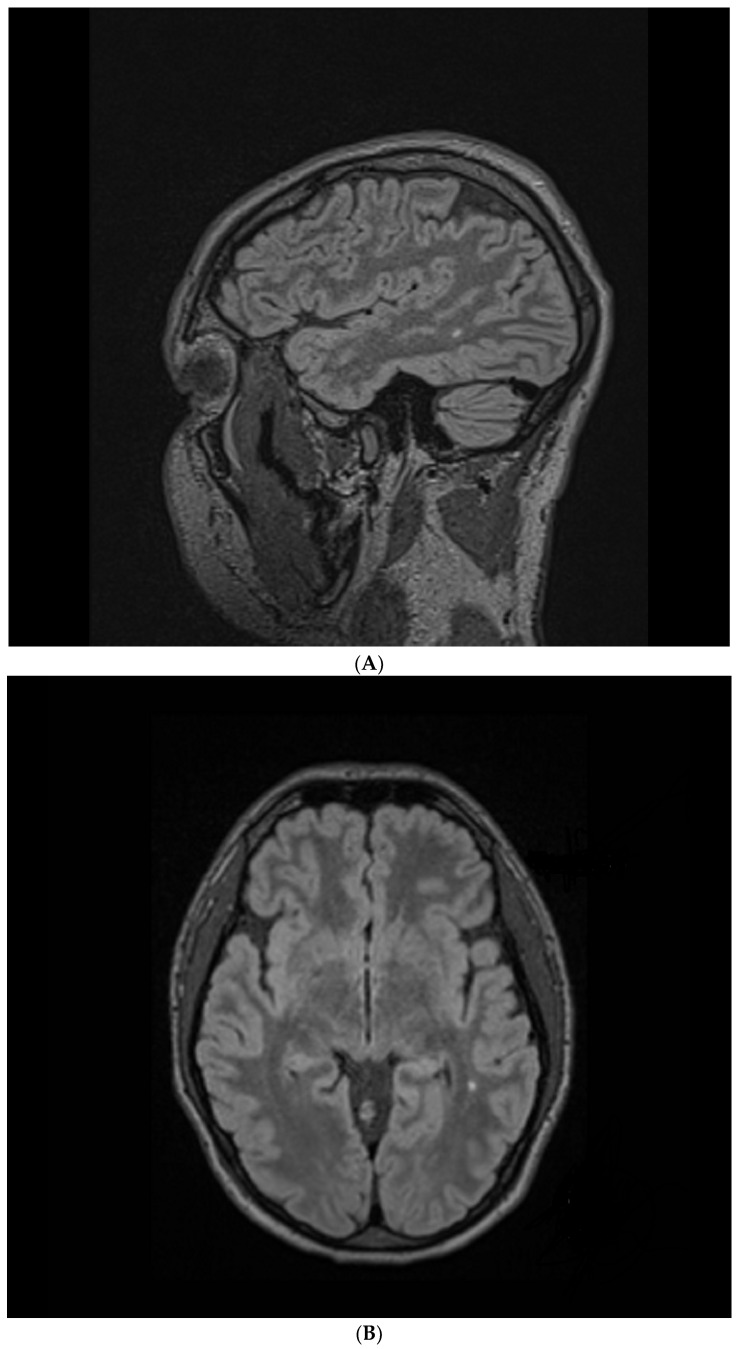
Anatomic magnetic resonance imaging (MRI) scans, using a 1.5 Tesla Siemens Avanto, without contrast. Repetition time (TR) = 6000 ms, echo time (TE) = 35.8 ms, matrix = 256 × 218 mm, number of excitations (NEX) = 186. (A) Sagittal Flair ISO, field of view (FOV) = 227 × 260 mm, slice thickness (SL) = 1.00 mm. (B) Axial Flair MPR, FOV = 260 × 260 mm, SL = 1.10 mm.
